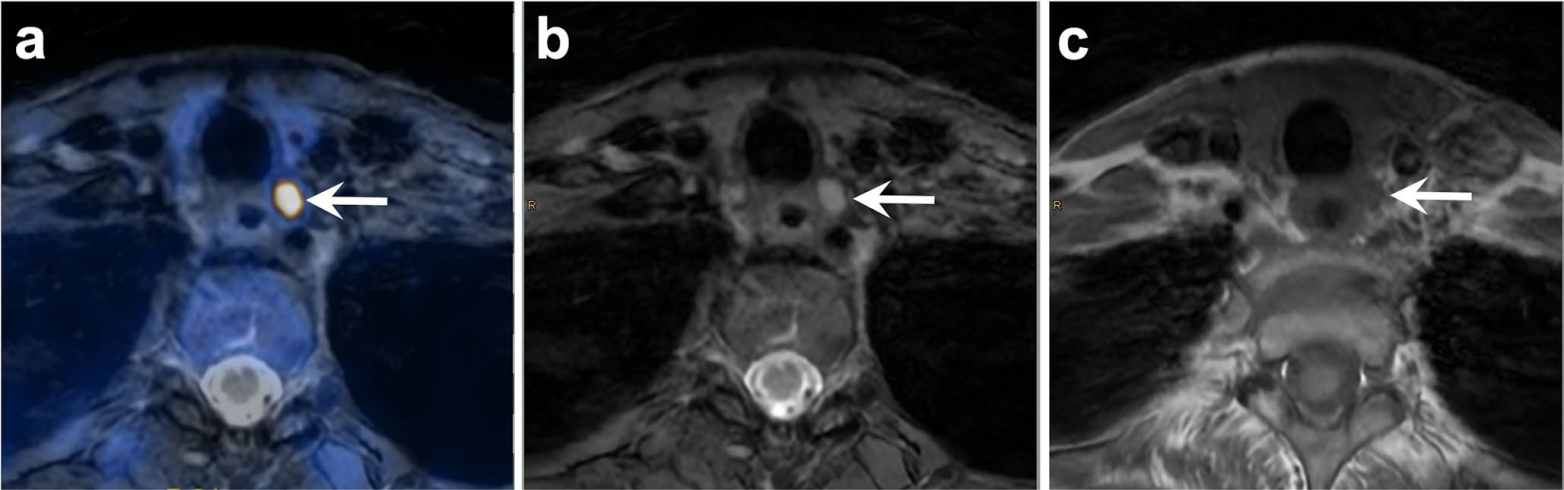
56 year-old woman with pHPT (PTH level 125.1 pg/mL, albumin-corrected serum calcium level 2.65 mmol/L). A (slightly overly) descended left-sided upper pole parathyroid adenoma (arrow; SUV_max_ 19.6, 7.5 x 5.5 x 15.0 mm) is seen on axial fused [^18^F]fluorocholine PET/MR image (a), axial T2-weighted flex water MR image (b) and axial T1-weighted FSE MR image (c)
